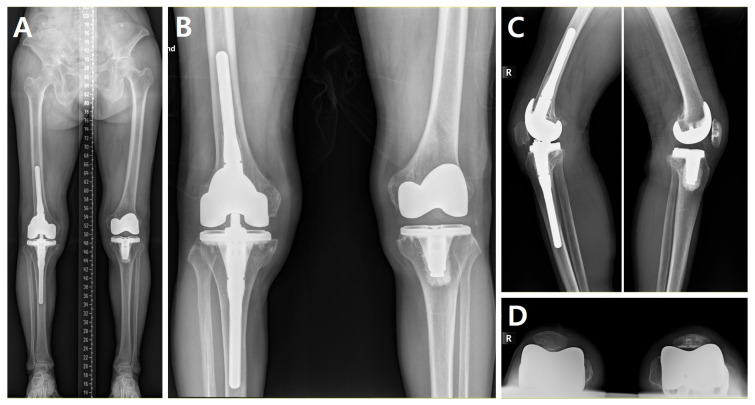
(A–D). Postoperative plain radiographs (Cases No. 1 and No. 2): (A) long-leg standing anteroposterior view; (B) standing anteroposterior view; (C) lateral view of right and left knees; and (D) skyline view.
